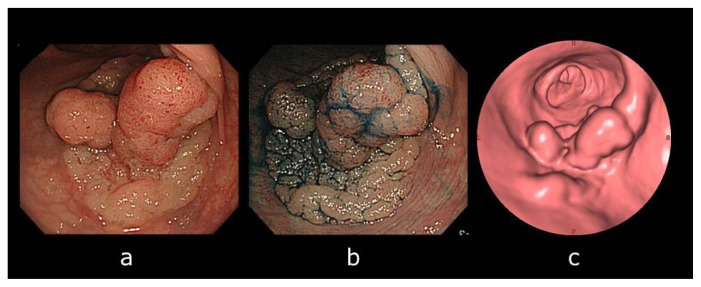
A laterally spreading tumor (LST) observed by computed tomographic colonography (CTC) in a 75-year-old man. (a) Colonoscopy revealed a Is + IIa (LST granular type: LST-G), 55 mm in diameter located in the rectum; (b) The lesion became apparent after indigo–carmine dye spraying; and (c) The surface endoluminal virtual image revealed the lesion on CTC. Endoscopic submucosal dissection (ESD) was subsequently performed on this LST-G lesion. Pathological diagnosis was a tubular adenoma with high- and low-grade dysplasia.
